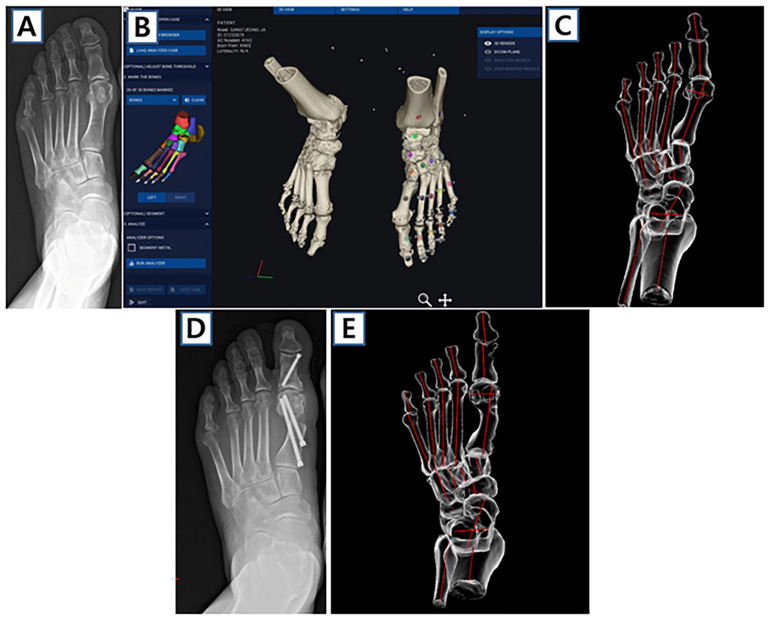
Semi-automatic 3D analysis of hallux valgus deformity using weight-bearing computed tomography and software. (A) Preoperative weight-bearing anteroposterior radiograph shows moderate hallux valgus deformity. (B) Bone marking with manual registration using the software and (C) automated 3D analysis with bone axis. (D) Postoperative image after minimally invasive surgery and (E) 3D analysis with bone axis.
